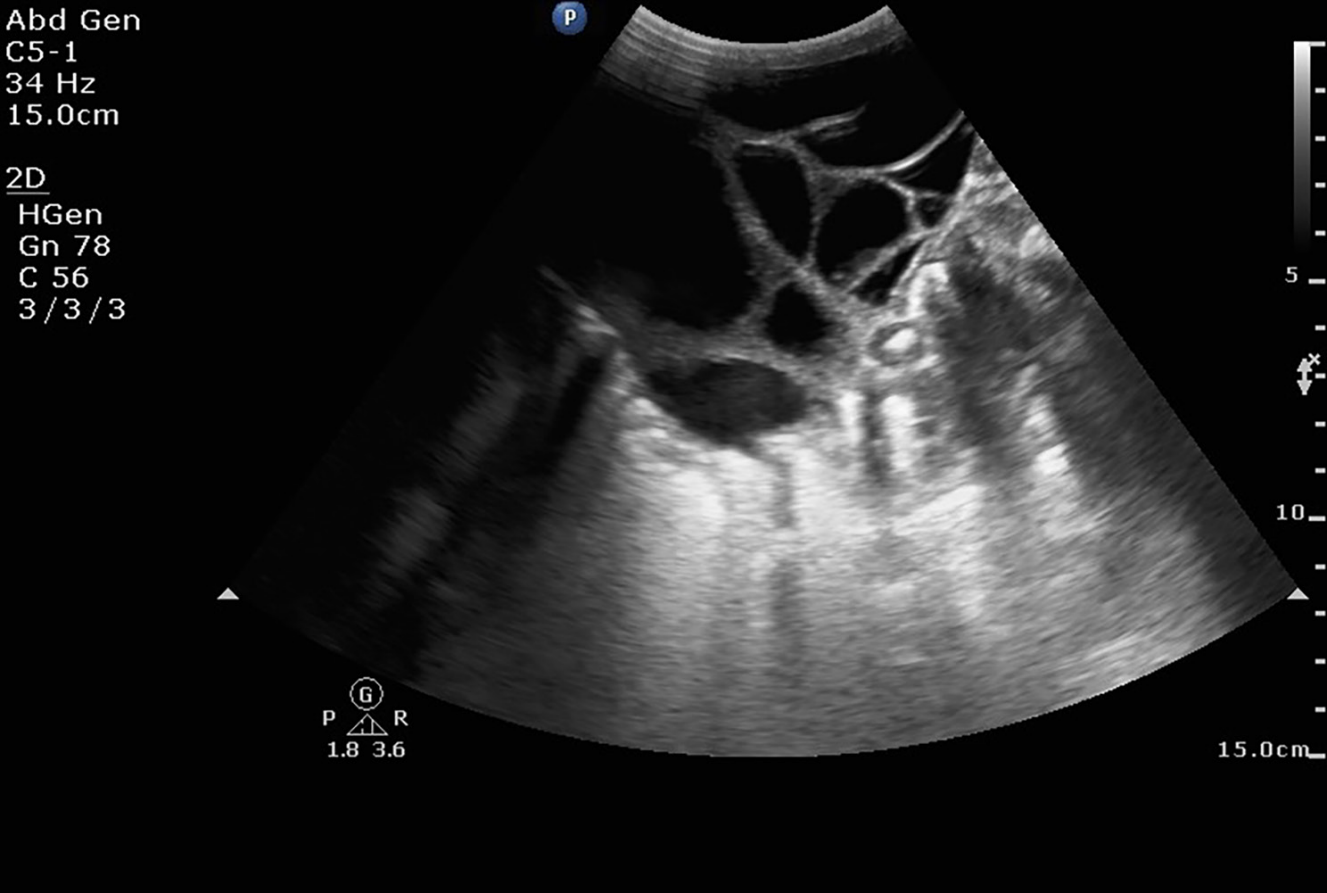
Presurgical abdominal ultrasound showing multiple cysts separated by setae was queried as multicystic dysplastic kidneys.
